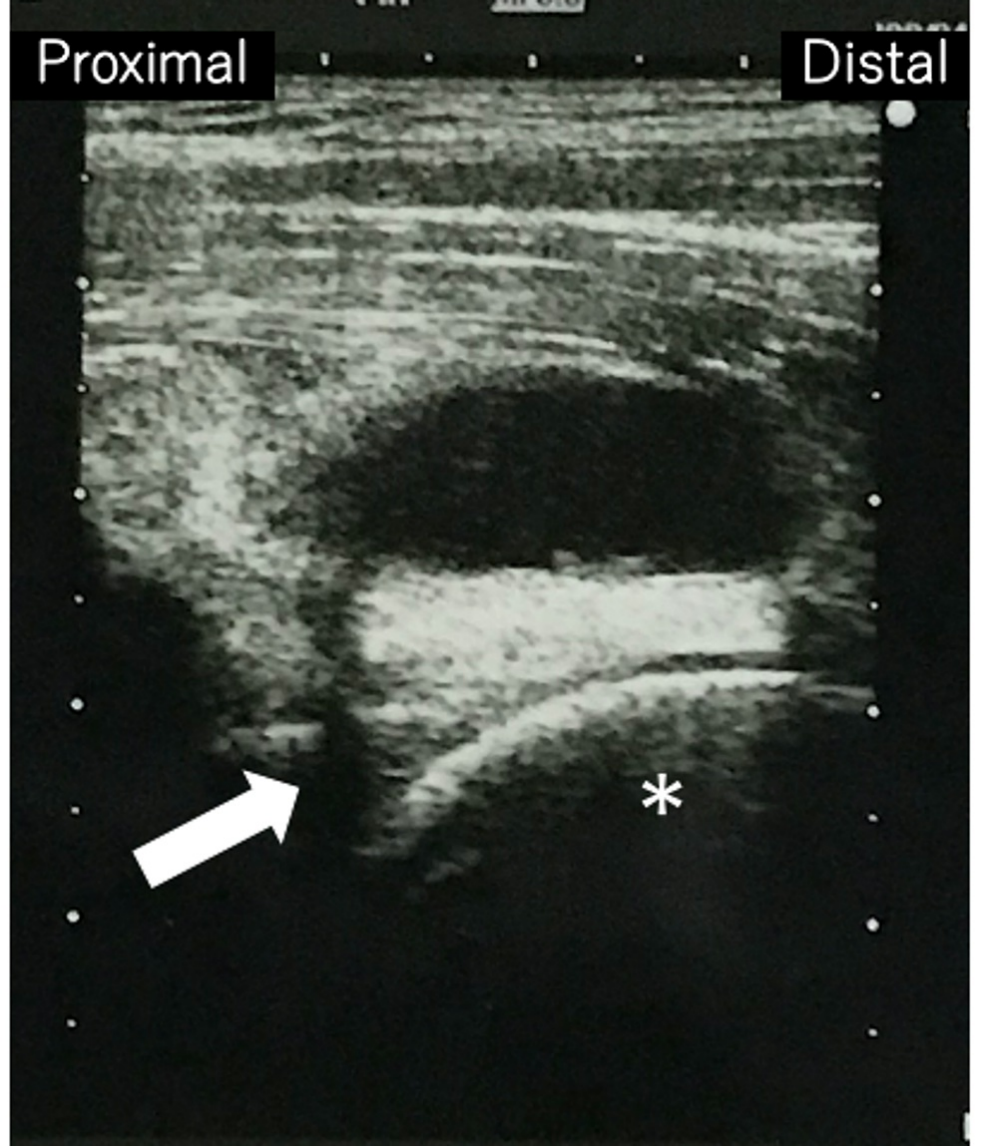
Preoperative ultrasonography image shows the hypoechoic mass anterior to the femoral head (asterisk) and its communication with the intraarticular space (arrow).
